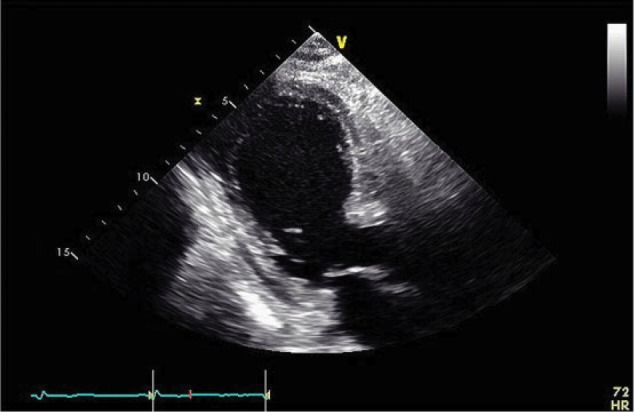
Parasternal long view on 2D transthoracic echocardiogram showing moderate ventricular septum and free wall thickness.
